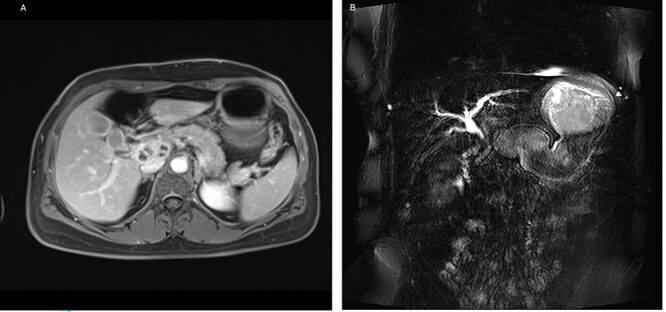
(A) Axial-T1w view with contrast: findings correlating with the CT and (B) magnetic resonance cholangiopancreatography showing a signal interruption of the CBD due to obstruction.
